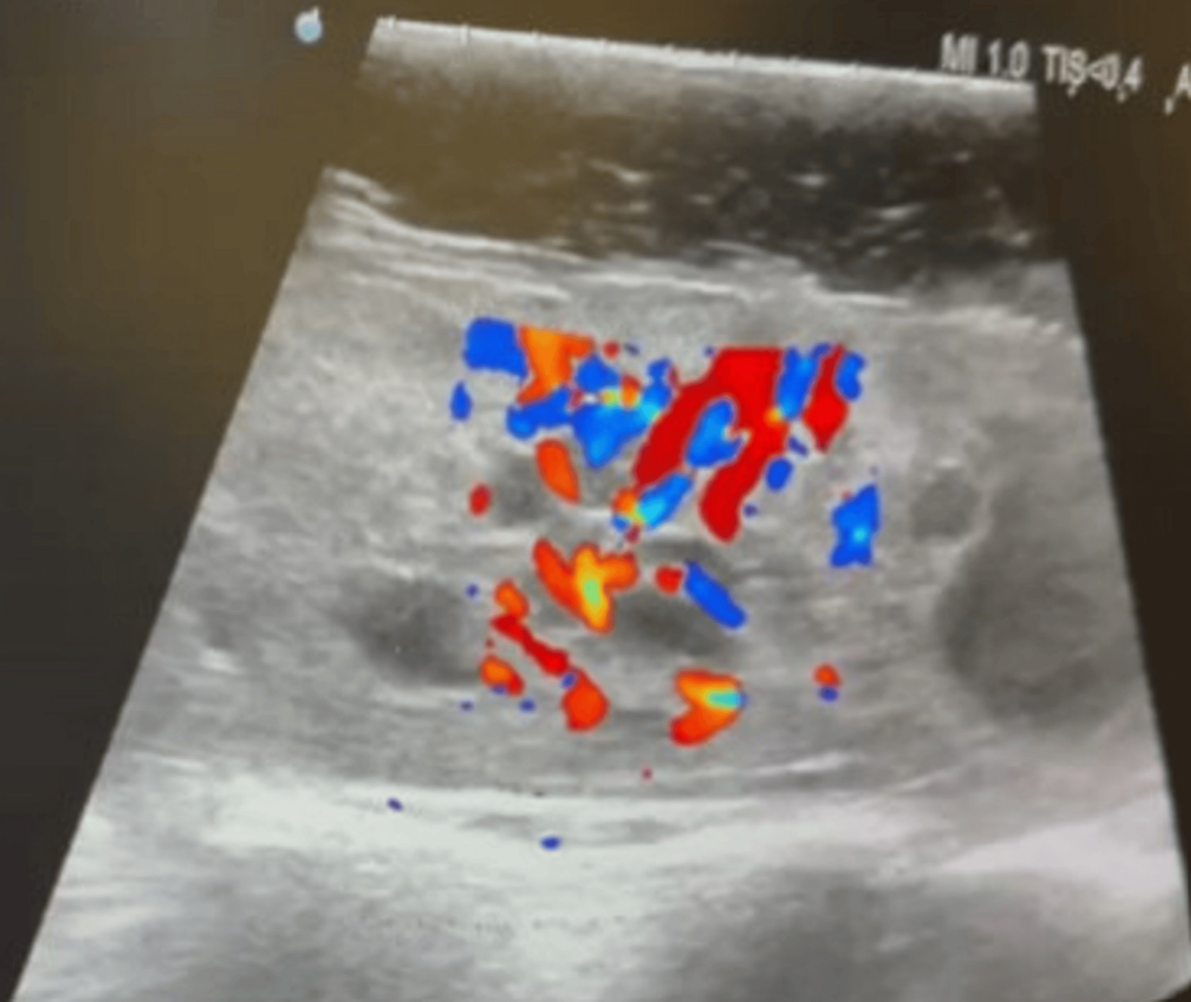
Intraoperative Doppler ultrasound at the surface of the dura revealing a highly vascular tumor
